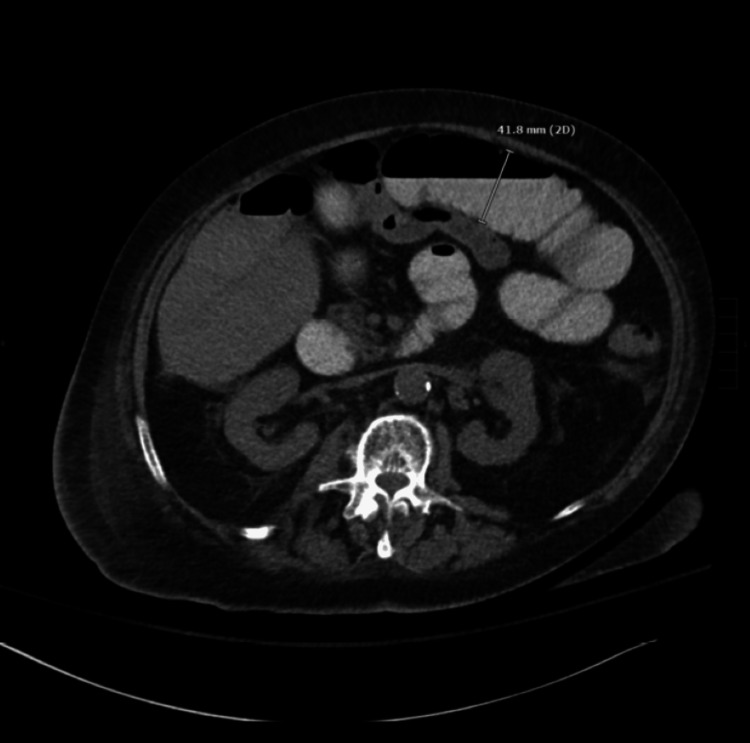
CT of the abdomen without contrast showing dilatation of the small bowel loops due to small bowel obstruction.
